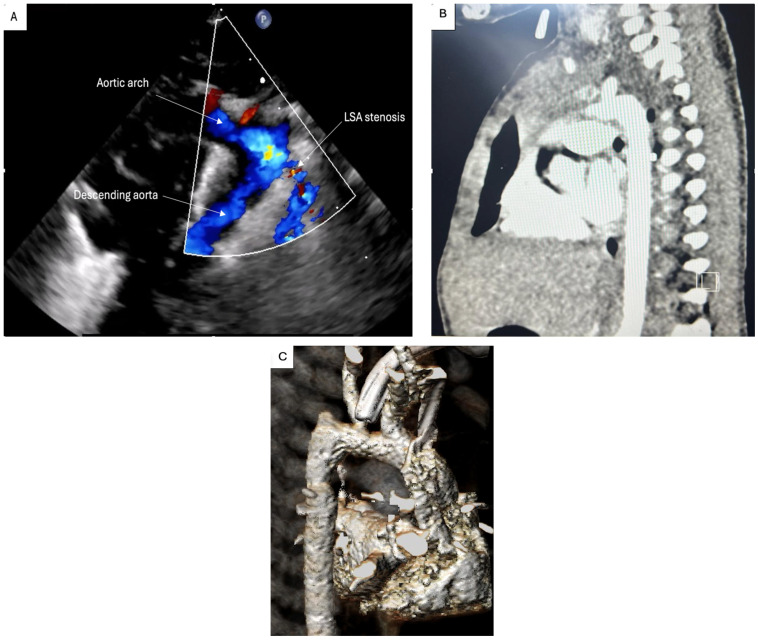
(A) Postoperative TTE showing the postoperative result of aortic coarctation repair and proximal left subclavian artery stenosis; (B) chest CT angiography showing the result of aortic coarctation repair; (C) postoperative 3D CT reconstruction; LSA: left subclavian artery.
